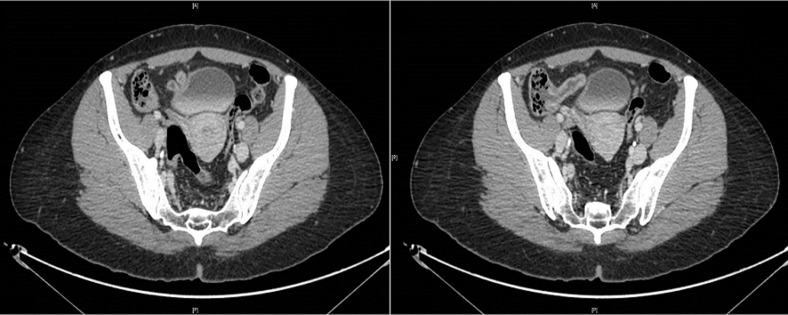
enhanced abdominal computed tomography scan (transverse images), showing the fistula between the ileocecal junction and the bladder, pointed by arrows
